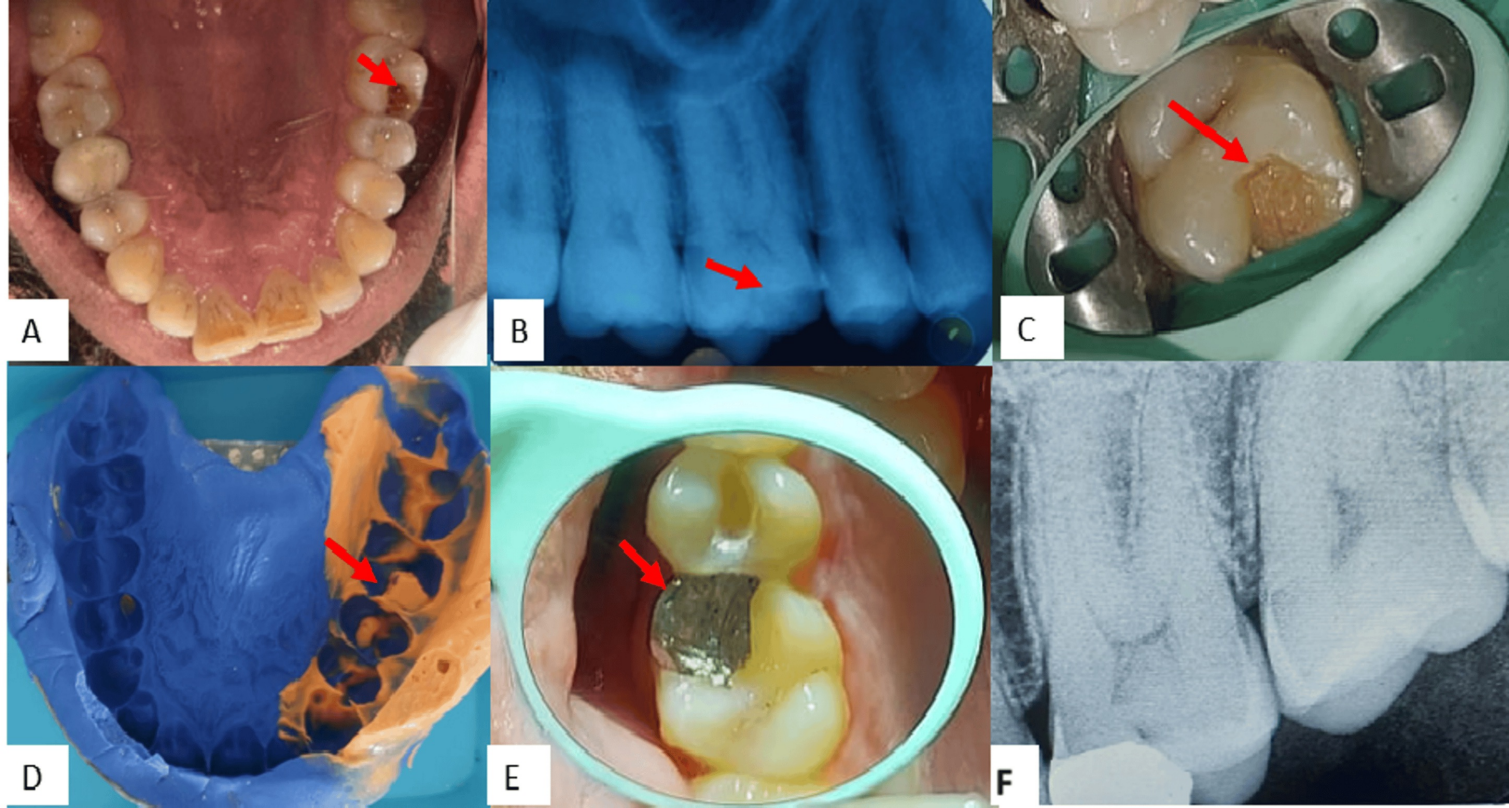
The image shows (A) preoperative intraoral, (B) preoperative radiograph, (C) cavity preparation, (D) elastomeric impression of the upper arch, (E) postoperative picture after cementation, and (F) postoperative radiograph
